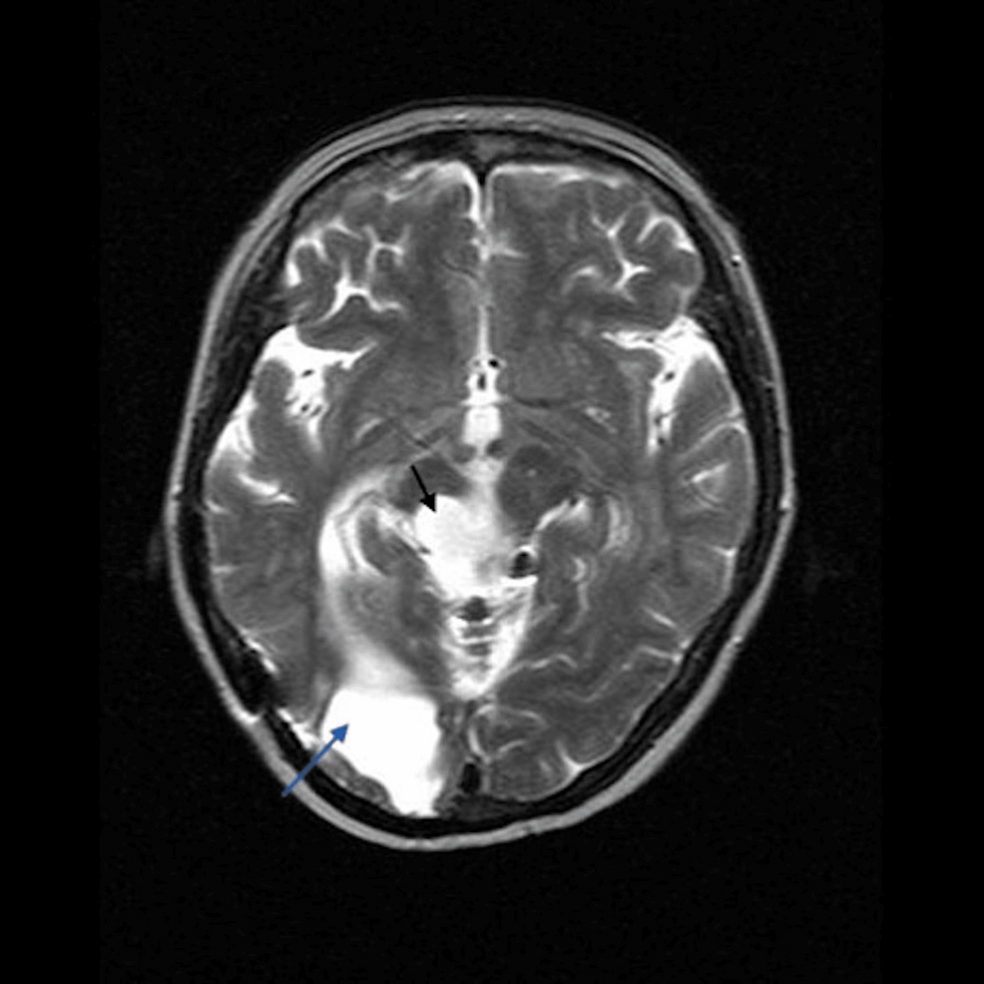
T2 weighted axial MRI showing cystic encephalomalacia in the right parietal/occipital region (grey arrow) in keeping with remote surgery for a glioblastoma, with additional post-surgical changes in the region of the midbrain (black arrow).
